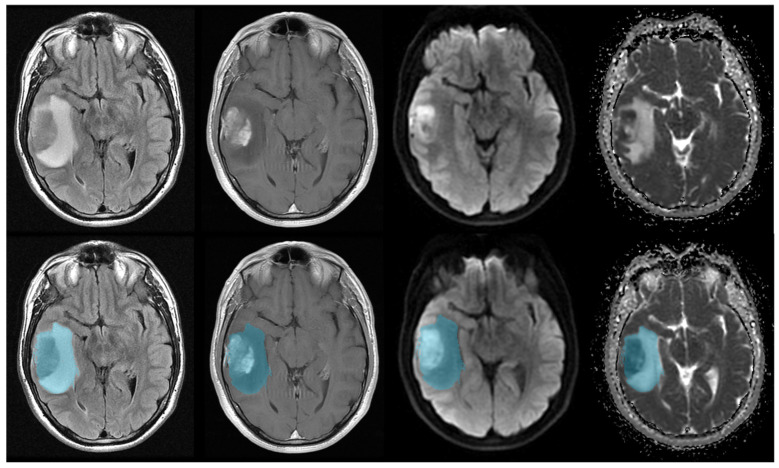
Manual segmentation of GBM in a representative patient. Top row: multisequence images (left to right: FLAIR, T1 post contrast, b1000, and ADC) through a single slice show GBM in the right temporal lobe. Bottom row: the tumor and surrounding T2 prolongation was manually segmented (shown in blue) on FLAIR images and overlaid onto other coregistered sequences.
